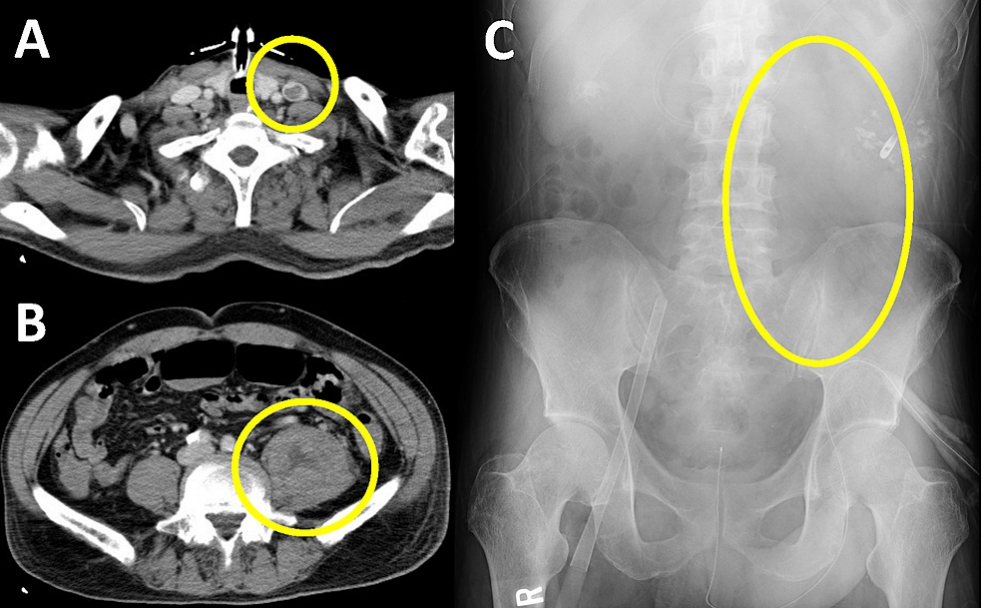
Computed tomography on day 20 and abdominal X-ray on day 14 (A) Enhanced computed tomography on day 20 showed left internal jugular thrombosis. (B) No extravasation was found in the left iliopsoas hematoma by the contrast media. (C) Abdominal X-ray on day 14 showed the appearance of the left psoas sign, suggesting correspondence to iliopsoas hemorrhage.
